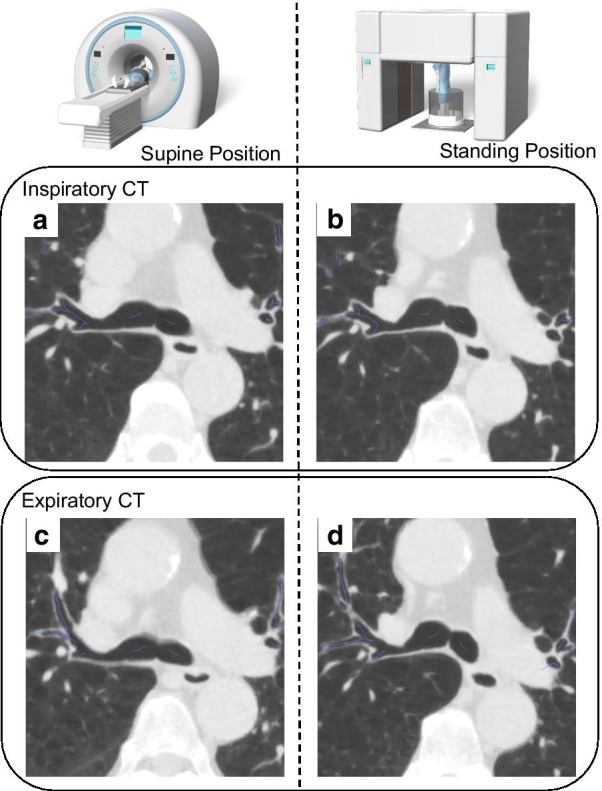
Axial CT images acquired at tracheal bifurcation level in a 74-year old male patient with COPD. a Inspiratory CT in the supine position. b Inspiratory CT in the standing position. c Expiratory CT in the supine position. d Expiratory CT in the standing position. The main bronchial areas were larger in the standing position than in the supine position on both inspiratory and expiratory CT. CT, computed tomography
